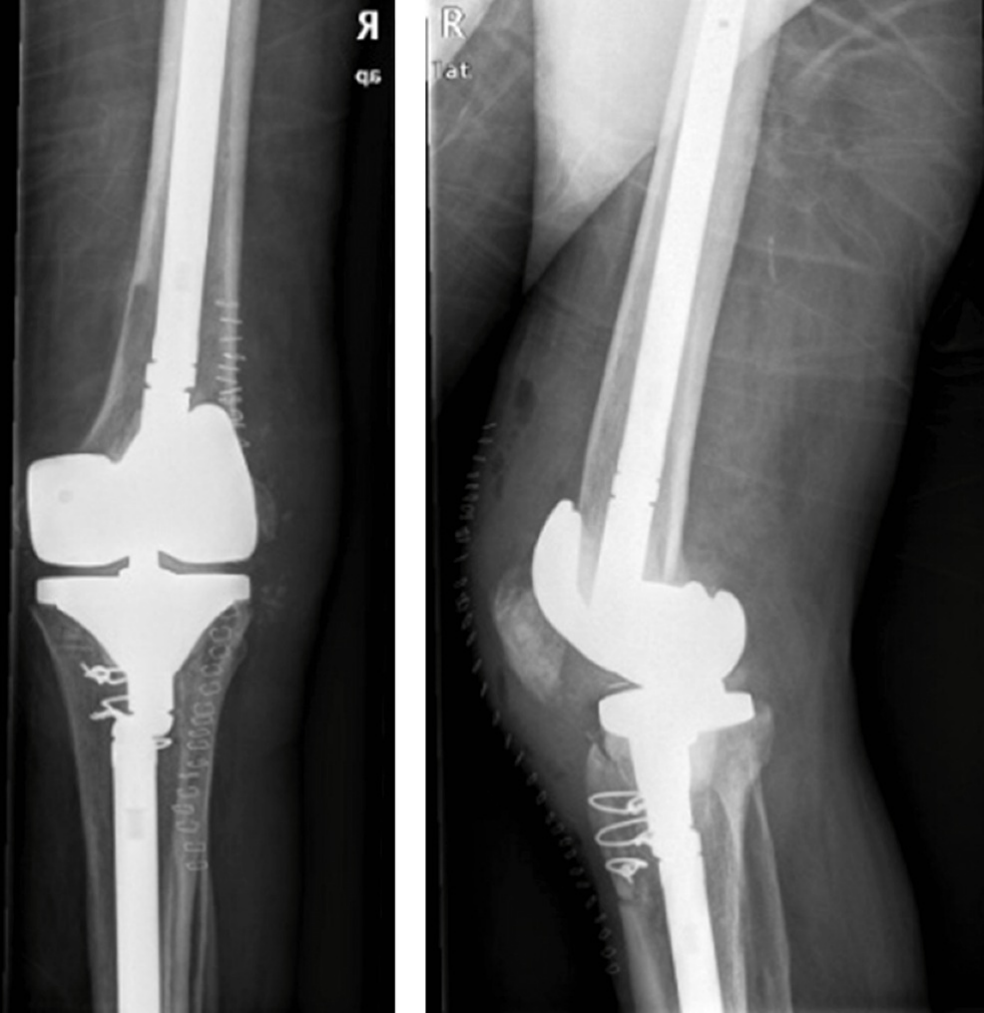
Anteroposterior and lateral X-rays after second stage operation.
